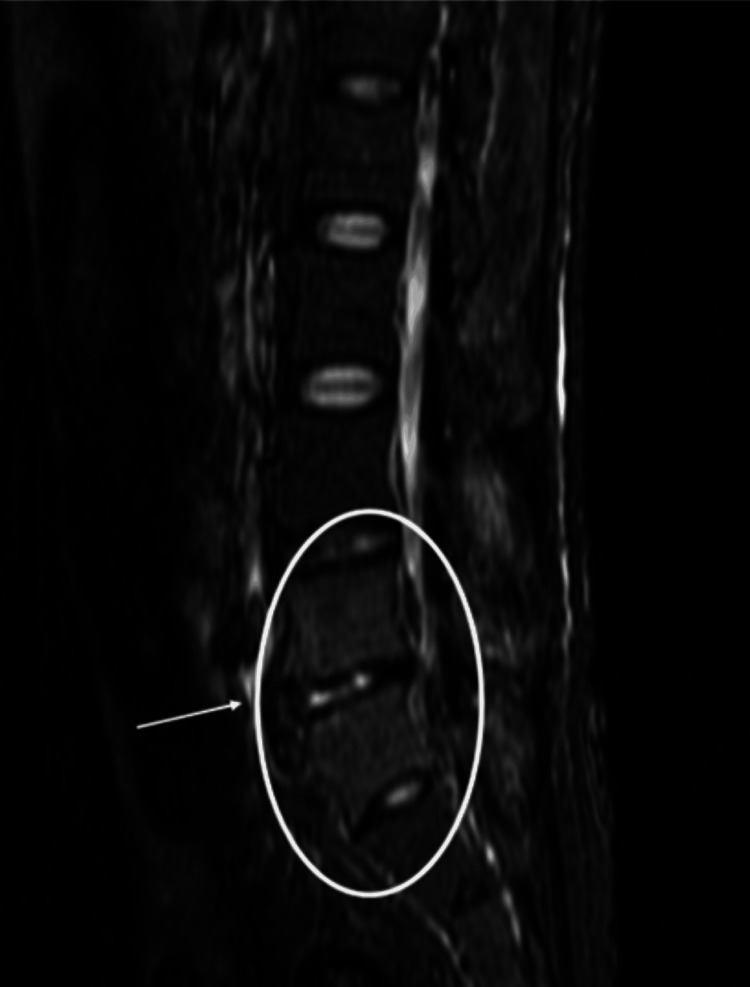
MRI of the lumbar region Coronal STIR MRI of the lumbar spine on day 9 of hospitalization. High signal intensity is observed around the intervertebral spaces between L4 and S1 (the circled area in the image), suggesting inflammatory changes consistent with early-stage pyogenic or reactive spondylitis. The L5 vertebral body is congenitally absent. These findings prompted CT-guided biopsy on day 17. STIR: short tau inversion recovery, MRI: magnetic resonance imaging, CT: computed tomography
